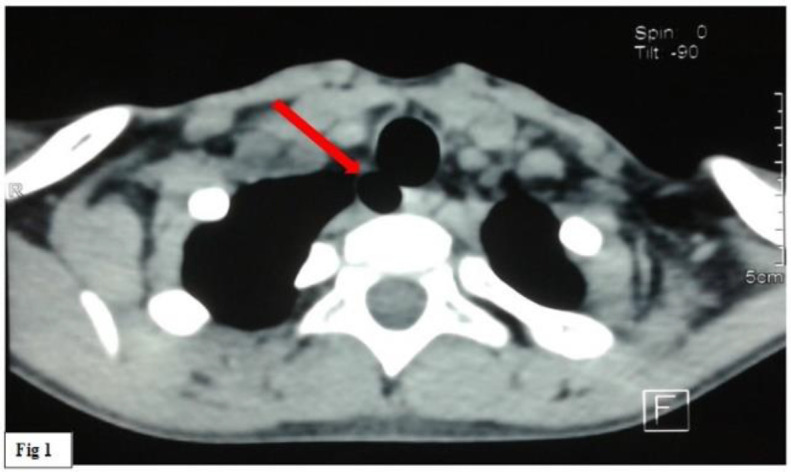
Cervical CT (computed tomography) scan in axial section shows a 11 mm air image located in the right posterolateral tracheal wall, at the thoracic inlet. It communicates with the tracheal lumen
